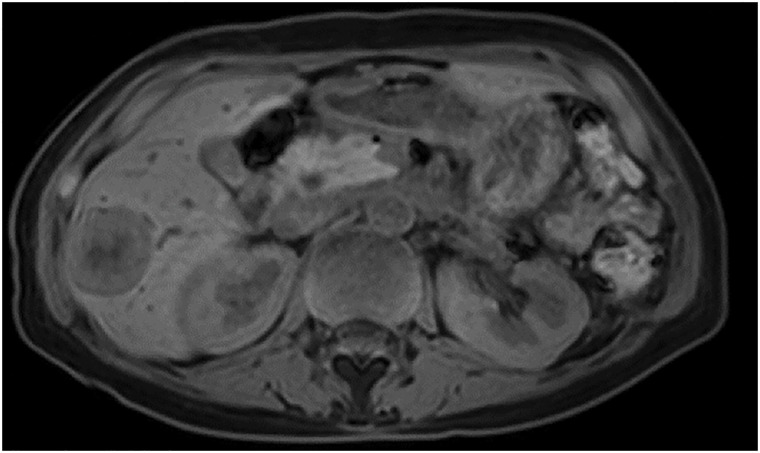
Late-phase Gd-EOB-DTPA-enhanced MRI of the S6 metastatic liver tumor after 3 courses of chemotherapy, showing a partial response. Gd-EOB-DTPA, gadolinium ethoxybenzyl diethylenetriamine pentaacetic acid
